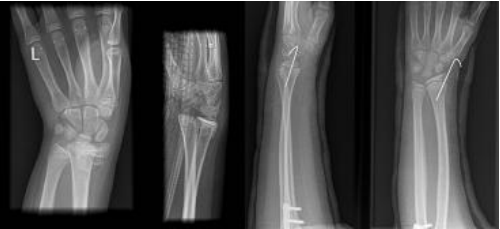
Radiographs showing a Salter-Harris type II distal radius fracture before and after wiring.
